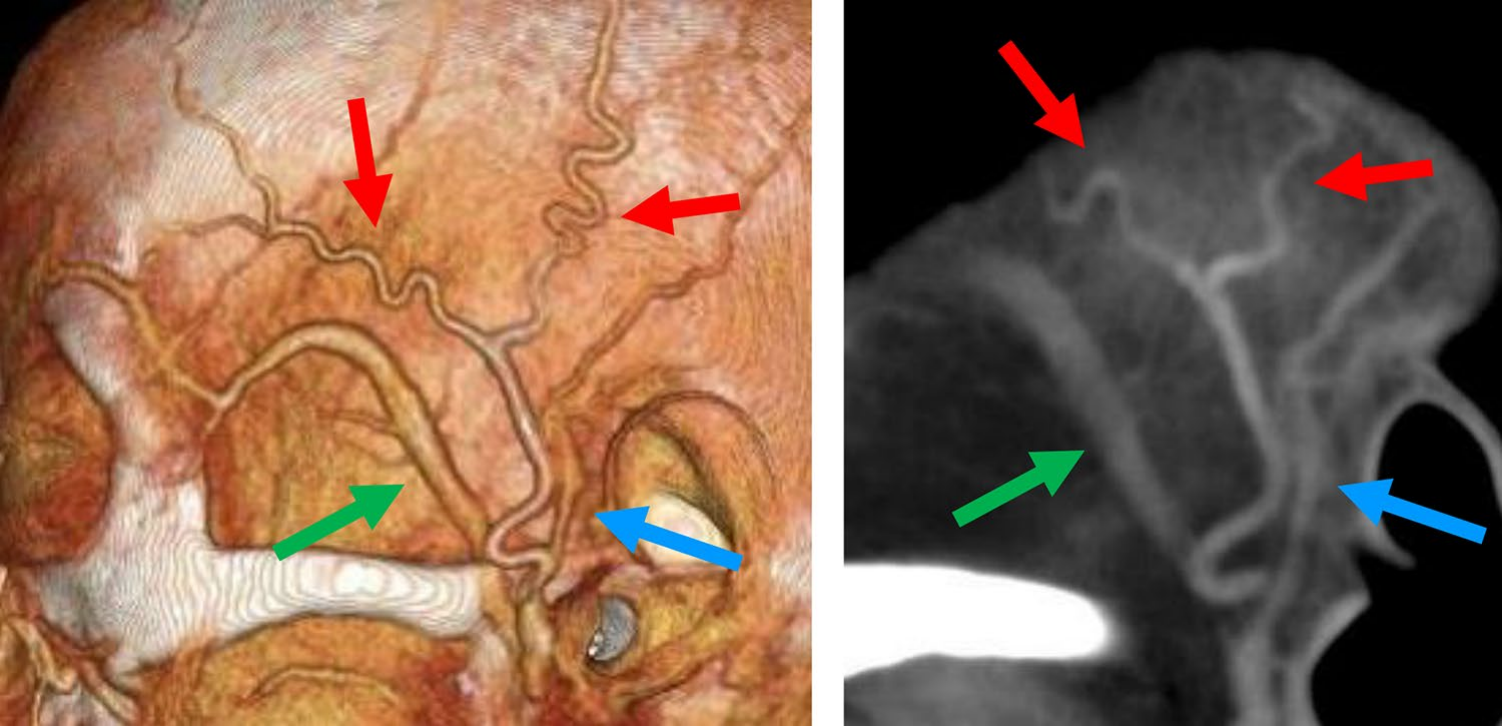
Anatomy of the temporal artery and veins. CT-angiography (A) and oblique sagittal reformat of unenhanced CT (B). Note the tortuosity and wide branching angle of the temporal artery (red arrows) which can be recognized on sagittal image (C) and distinguished from the middle temporal (green arrow) and superficial temporal (blue arrow) veins
